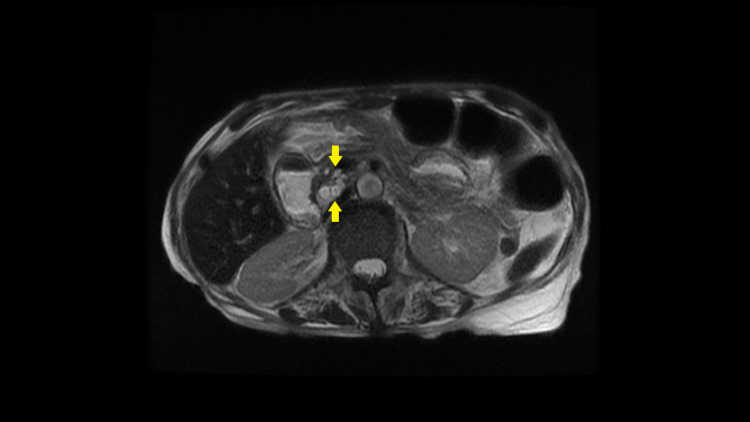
T1-weighted MRI scan of the abdomen with intravenous contrast, axial view, in a 66-year-old female presenting with four days of abdominal pain and nausea/vomiting Axial view of an MRI scan of the abdomen with intravenous contrast demonstrates a 1.7 x 1.4 x 2.0 cm cluster of multi-lobulated cystic lesions (yellow arrows), indicative of a side-branch intraductal papillary mucinous neoplasm (IPMN), abutting the medial/posterior aspect of the pancreatic head and neck.
